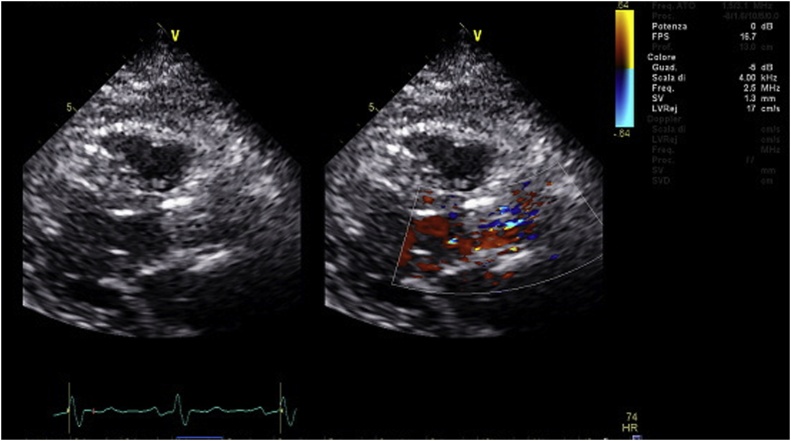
Post operative echocardiography shows correct position of the Perceval sutureless valve with no para-valvular leaks.
